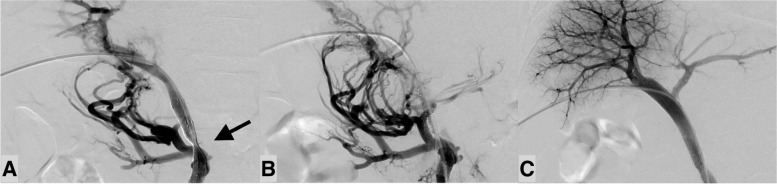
A patient with residual stenosis (in-stent) which resulted in stent occlusion. A man in his 70 s presented with hematochezia eight months after pancreaticoduodenectomy for pancreas cancer. Portal vein (PV) occlusion due to postoperative pancreatic fistula was confirmed on contrast-enhanced computed tomography (CT). In addition, development of hepatopetal collateral veins were observed around the choledochojejunostomy. Portography showed PV occlusion with hepatopetal collateral vein development. Two stents (SMART Control; Cordis, Hialeah, FL, USA) were placed through the occlusion and post-stent balloon dilatation was performed up to the nominal pressure. A Although residual in-stent stenosis (arrow) remained, PV flow improved at the end of the procedure. Contrast-enhanced CT was performed two days after stent placement and intra-stent thrombus was suspected. His hematochezia still persisted and anemia progressed, so additional treatment was scheduled. B Portography four days after the first stent placement shows recurrent PV occlusion. C After additional stent placement (SMART Control), hepatopetal PV flow had improved and flow through the collateral veins disappeared. The stent patency has been maintained for six years after the placement
